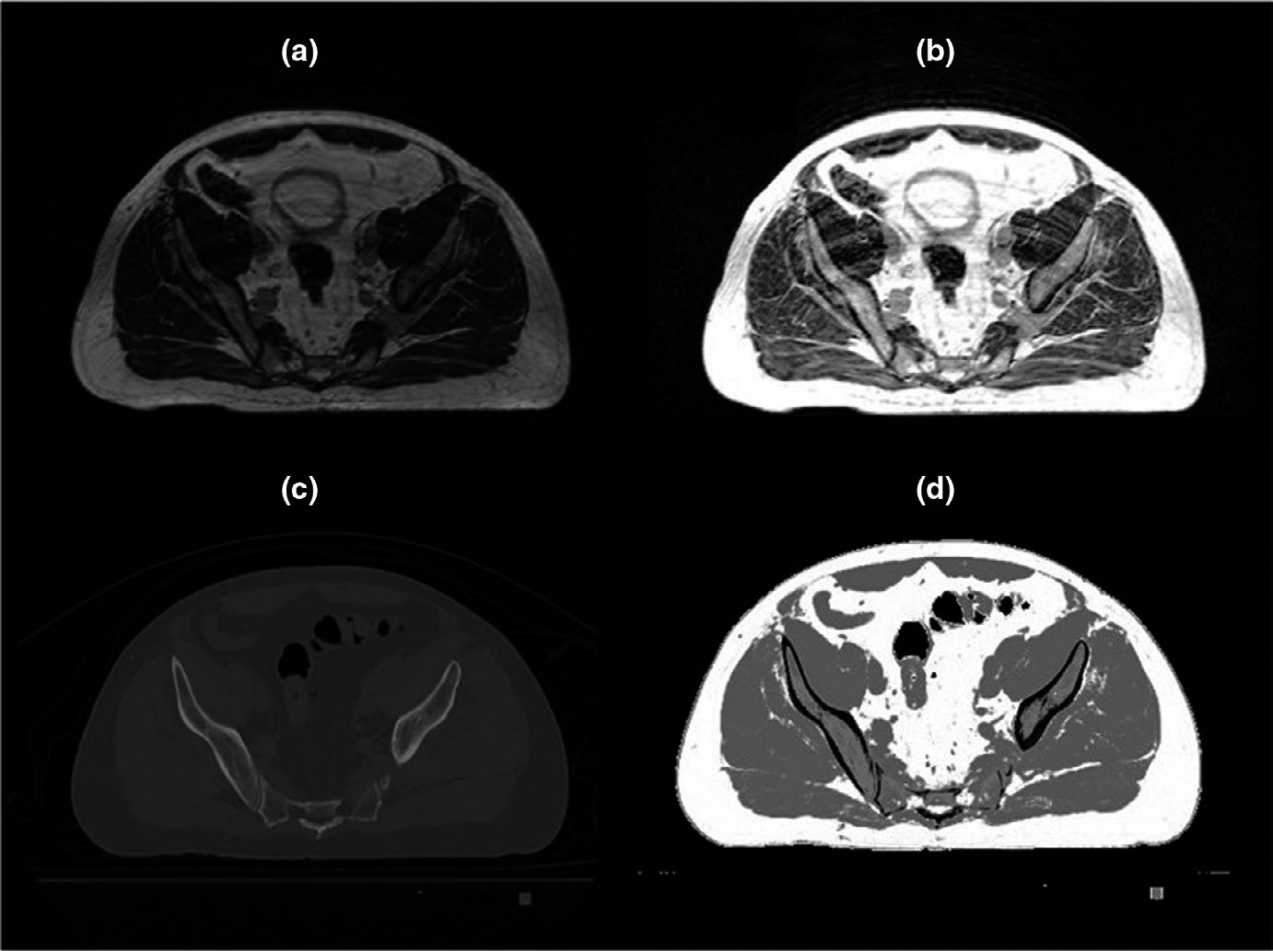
Image pre‐processing steps performed prior to deforming planning CT to TRUFI MRI image. (a) original TRUFI MRI, (b) standardized TRUFI MRI, (c) planning CT, and (d) intensity‐matched CT
